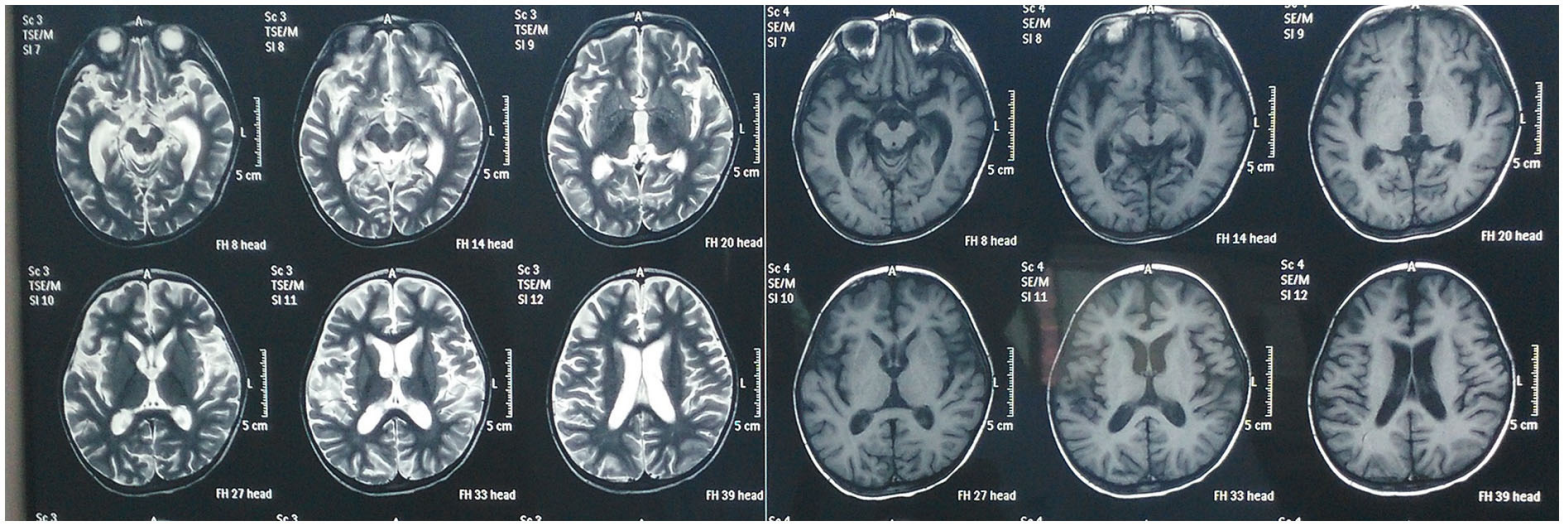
Magnetic resonance imaging of the brain before receiving Tongdu Xingshen acupuncture showed mild brain atrophy and pachymeningeal enhancement associated to meningitis.
